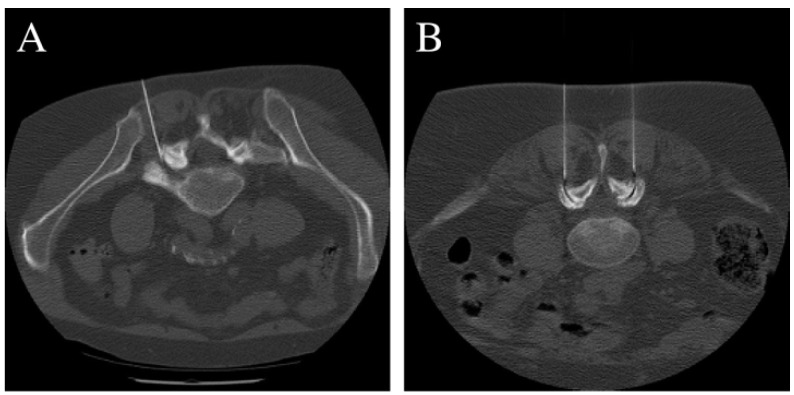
Computed tomography showing the positioning of the spinal needle(s) lateral to the midline at the level of the affected nerve root via an extraforaminal approach at the L5/S1 neuroforamen (A) and L4/5 facet joint (B).
